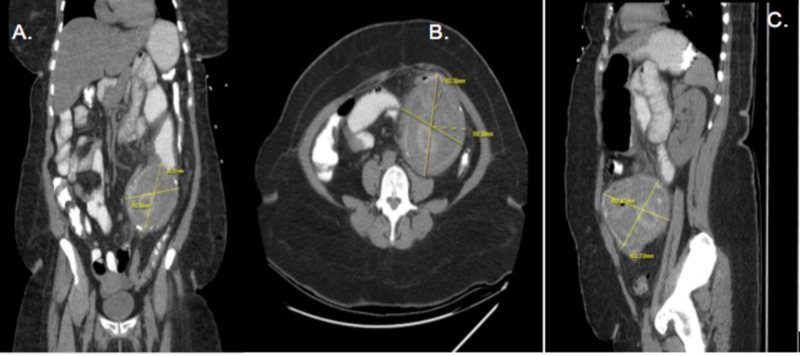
Computed tomography angiography (CTA) of the abdomen and pelvis with contrast showing complex mass with asymmetrical wall thickening, inflammatory stranding, and mild bowel distention (A) Axial view 10.33 cm x 10.03 cm, (B) Coronal view 10.3 cm x 9.32 cm and (C) Sagittal view 10.28 cm x 10.27 cm.
